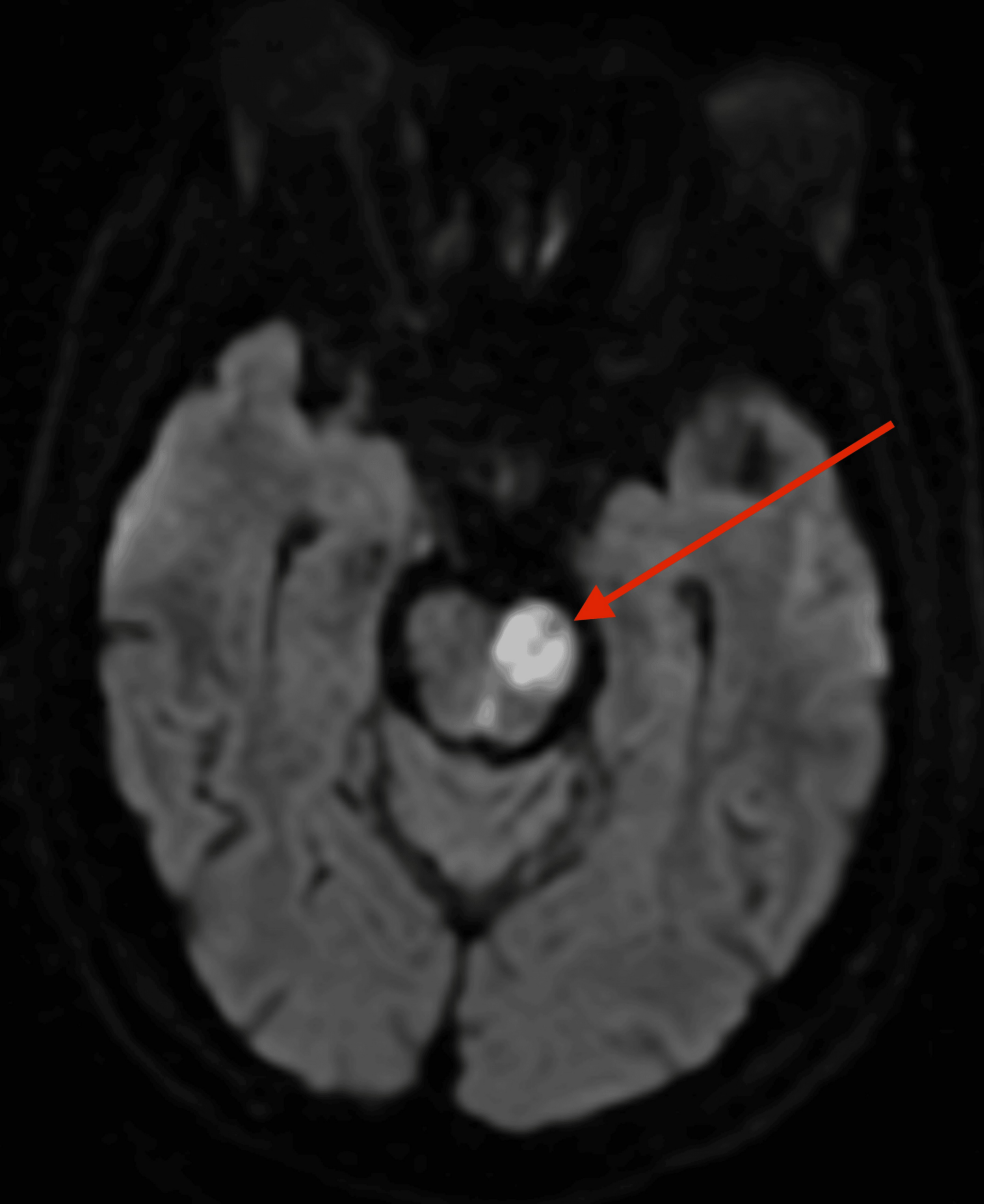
MRI Brain showing left pontine infarct MRI brain showing an area of restricted diffusion in the left anterior pons (indicated by red arrow), consistent with acute ischaemia. Findings are in keeping with a left pontine infarct.
